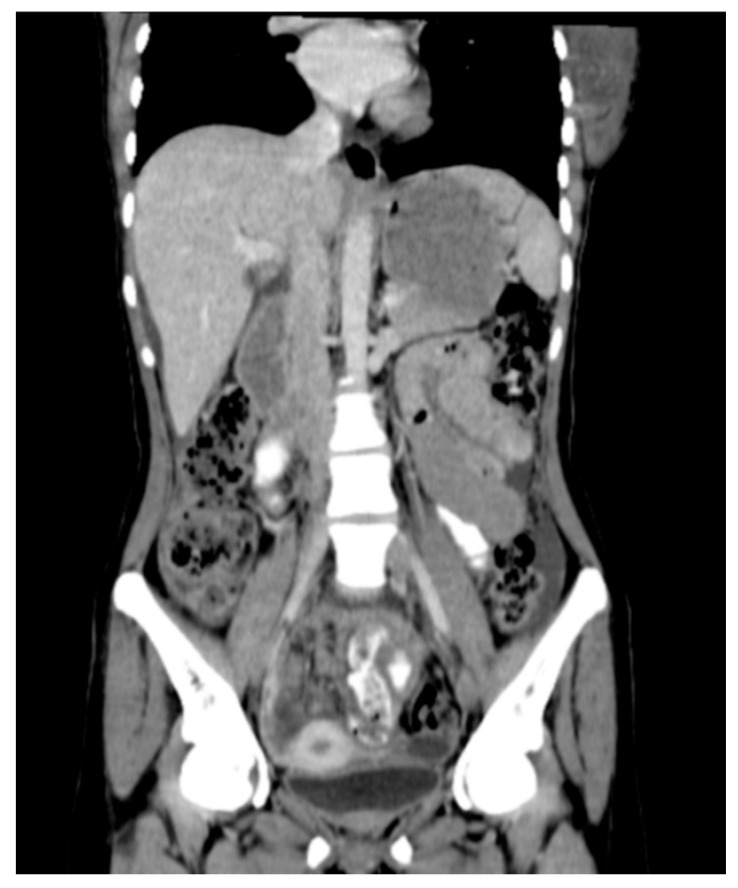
Contrast-enhanced coronal CT image of the abdomen demonstrating imaging features consistent with peritoneal TB, including peritoneal thickening and nodularity with associated ascites. These findings are characteristic of tuberculous peritonitis and may mimic peritoneal carcinomatosis. Case courtesy of Dalia Ibrahim, Radiopaedia.org, rID: 216518, licensed under CC-NC-BY-SA 3.0 [61].
